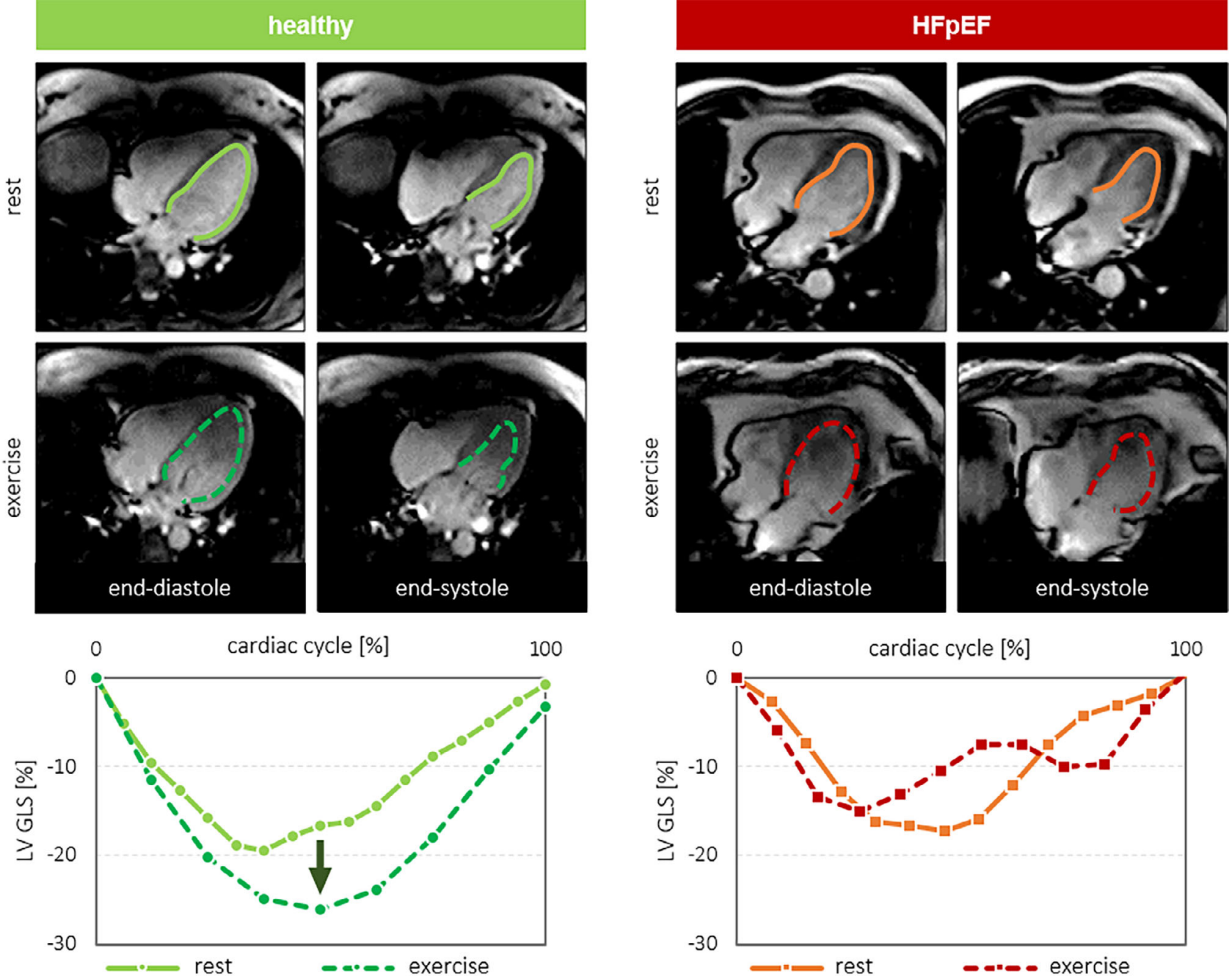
Example of how real‐time cine MRI during supine bicycling exercise stress can unmask myocardial dysfunction. Note that left ventricular (LV) global longitudinal strain (GLS) measured along the endocardial LV wall is augmented (green arrow) upon exercise in the healthy subject (male; 28 years). In the patient (male; 70 years) with heart failure with preserved ejection fraction (HFpEF), LV GLS appears normal at rest (orange), but fails to increase during exercise (red). Data courtesy of the Department of Radiology and Nuclear Medicine, Amsterdam University Medical Centers, Amsterdam, The Netherlands.
